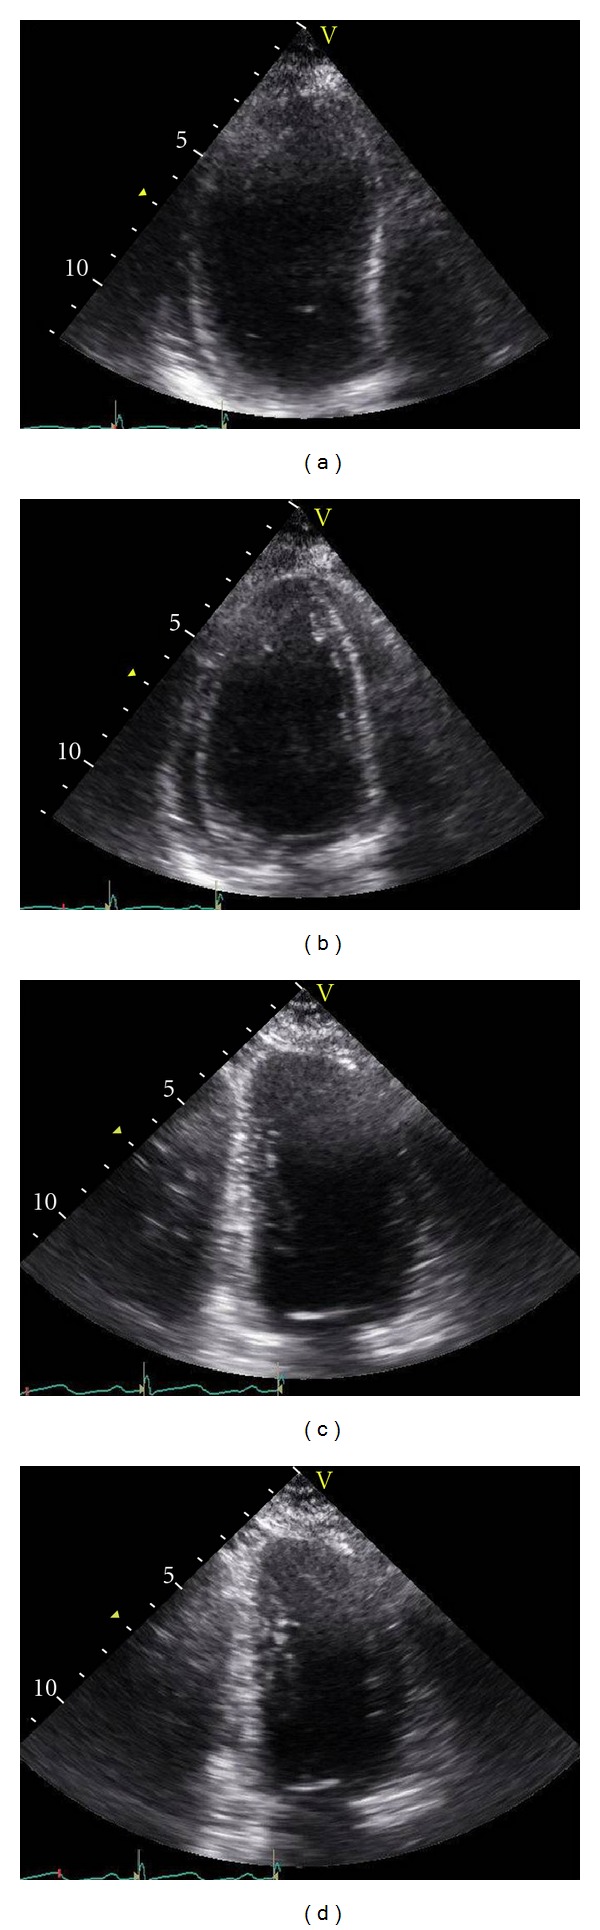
End-diastolic (a) and end-systolic (b) frames of two chamber view on initial echocardiography taken 12th day showing severe left ventricular systolic dysfunction with akinesia of the left ventricular base and mid-portion, and hypercontractility of the apex, and a followup echocardiography at the end of diastole (c) and systole (d) taken 28th days showing nearly normalized cardiac function without regional wall motion abnormalities.
